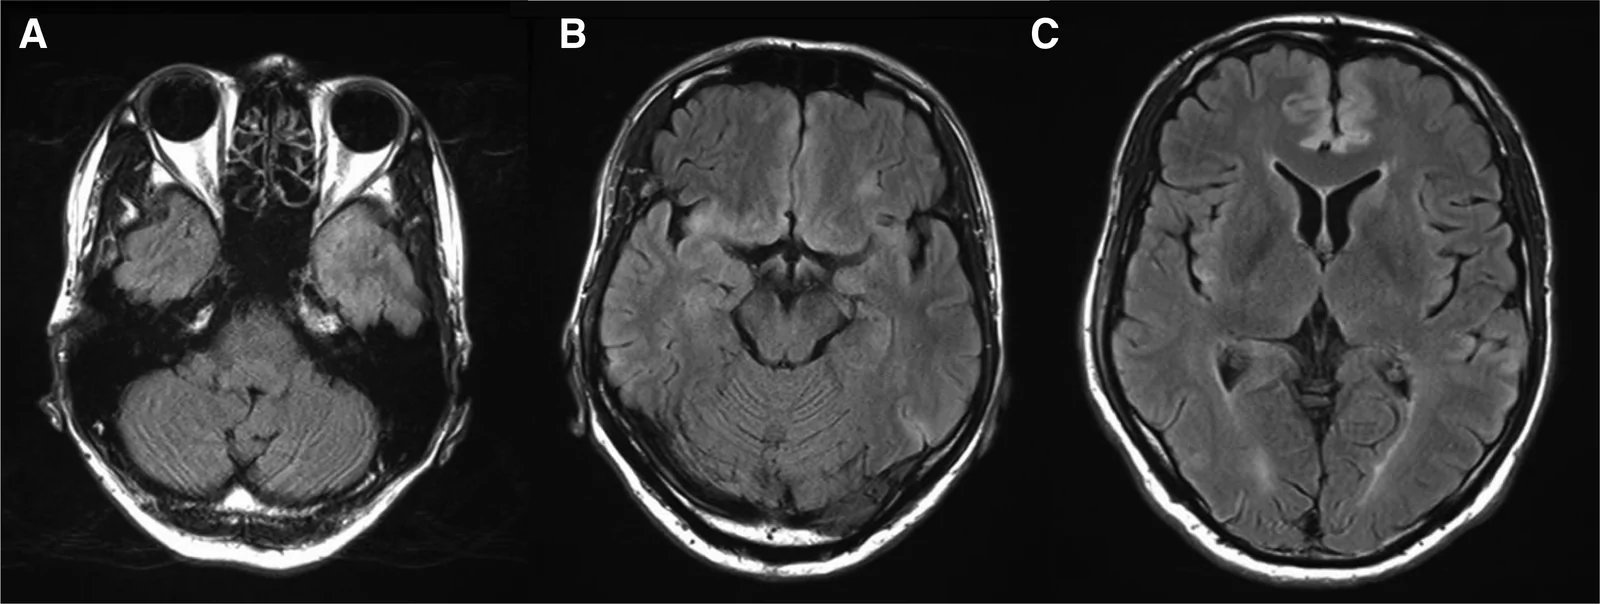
Brain magnetic resonance imaging on admission. Axial fluid-attenuated inversion recovery images (A–C) show hyperintense lesions in the extensive cerebral cortex.
